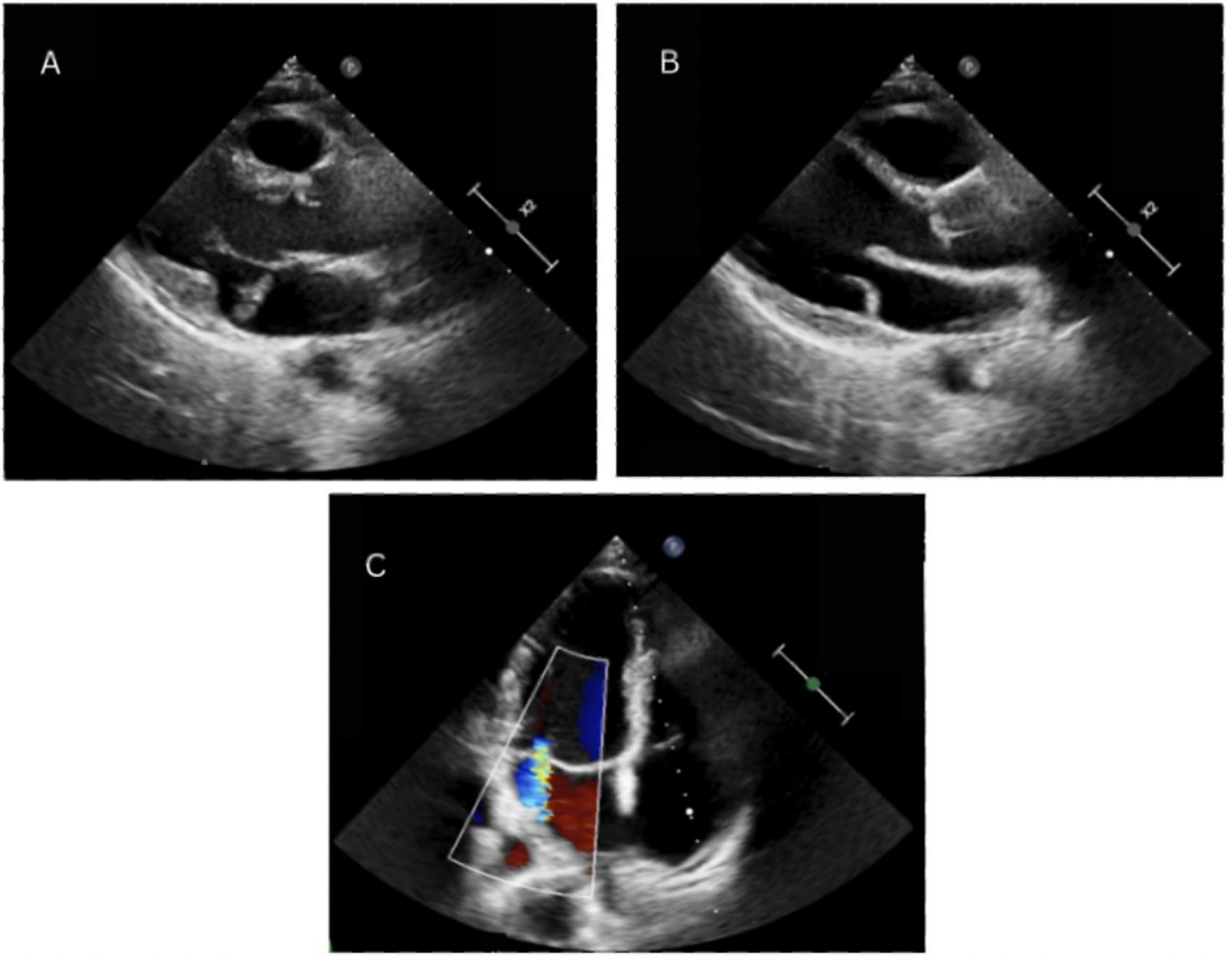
Echocardiography demonstrating mitral annular disjunction. Parasternal long-axis view obtained at end-systole and diastole, showing the separation between the posterior mitral valve leaflet insertion point and the left ventricular myocardium (A,B). Color Doppler imaging visualizing mitral regurgitation, evidenced by a central regurgitant jet (C).
